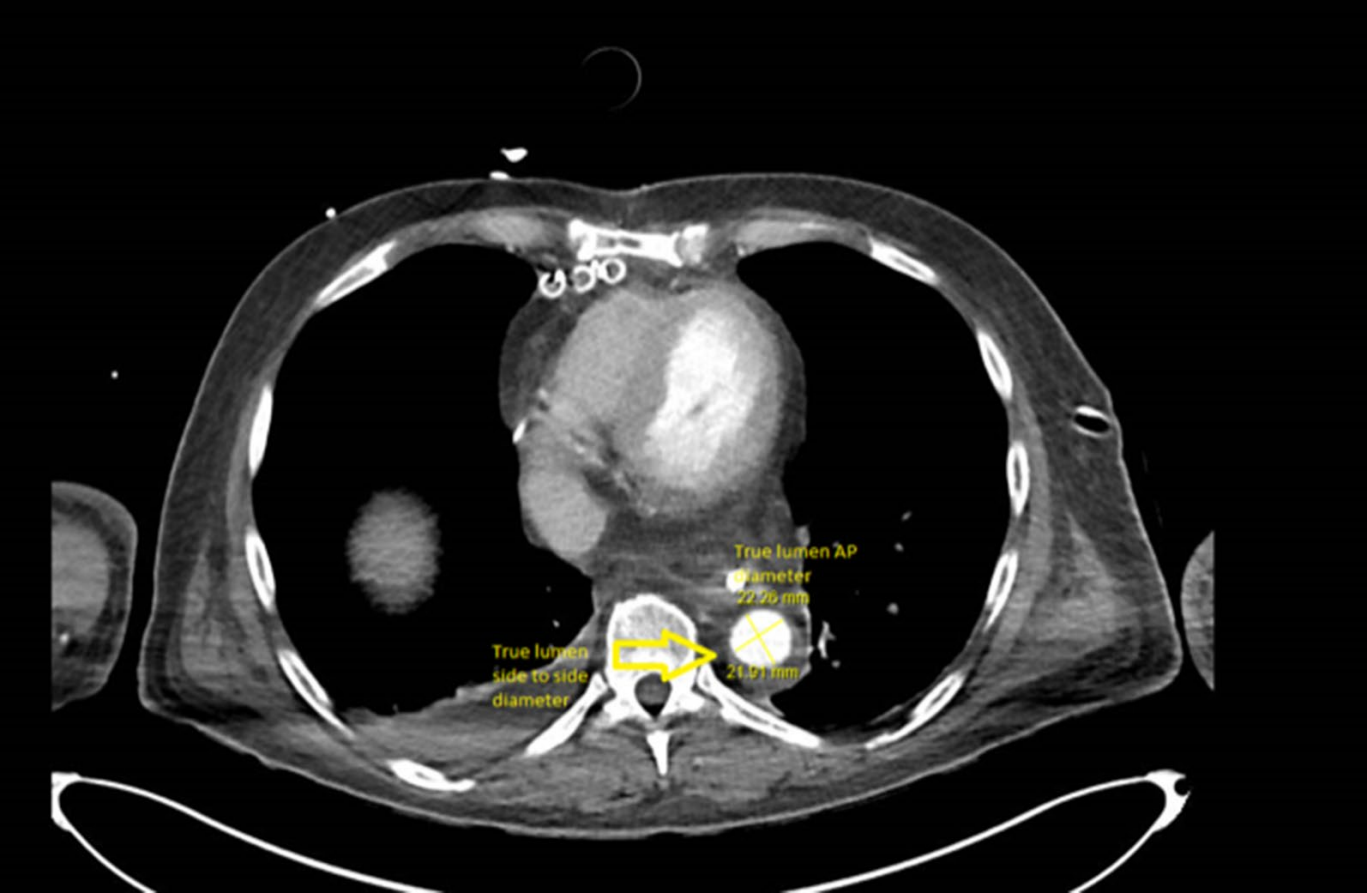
Multislice computed tomography showing true lumen largest antero-posterior and side to side diameters measured at the level of the right diaphragmatic dome
